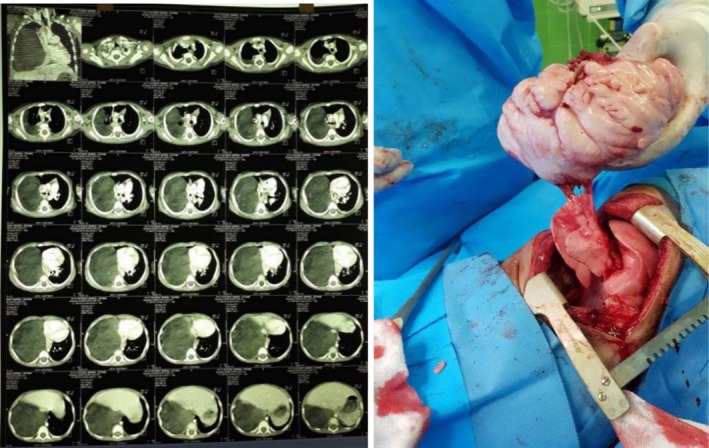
Left picture, lung CT scan of a 4‐year‐old boy with a huge intrathoracic mass. The right picture shows that the huge myolipoma mass was completely resected by our surgical method.
